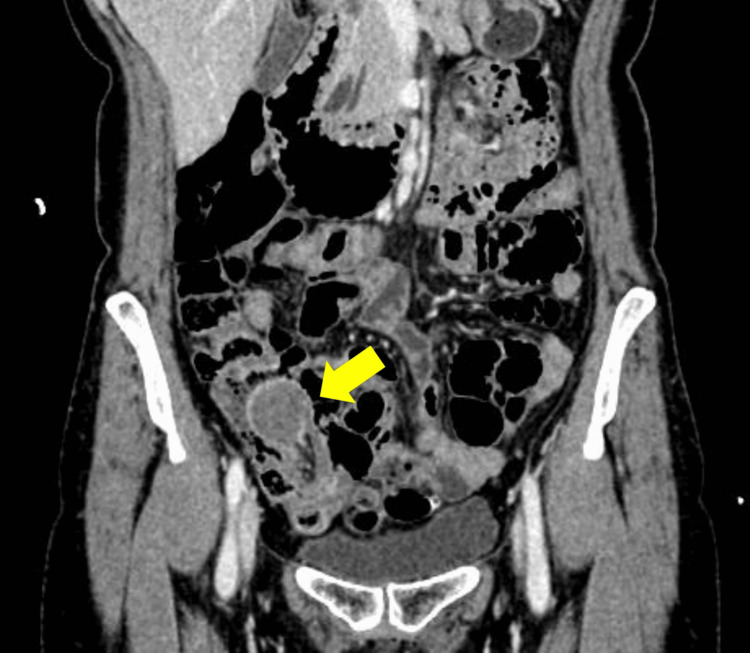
Contrast-enhanced CT (lead mass): A spherical mass (arrow) in the terminal ileum prolapsing into the ascending colon CT: computed tomography
